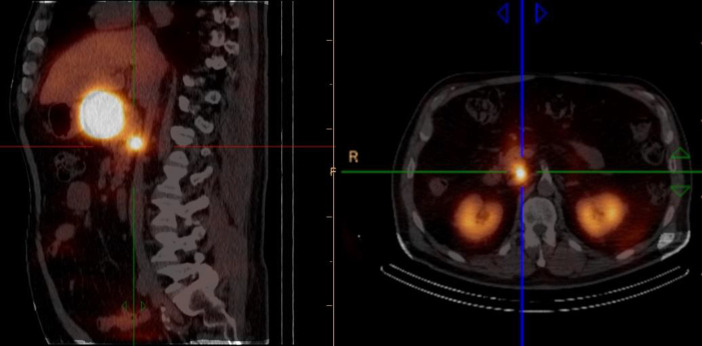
SPECT-CT of Case 2 after 740 MBq ^99m^Tc-Tektrotyd®. Transaxial and sagittal fused images demonstrate intense uptake in both the enlarged adenopathy and the primary duodenal lesion
